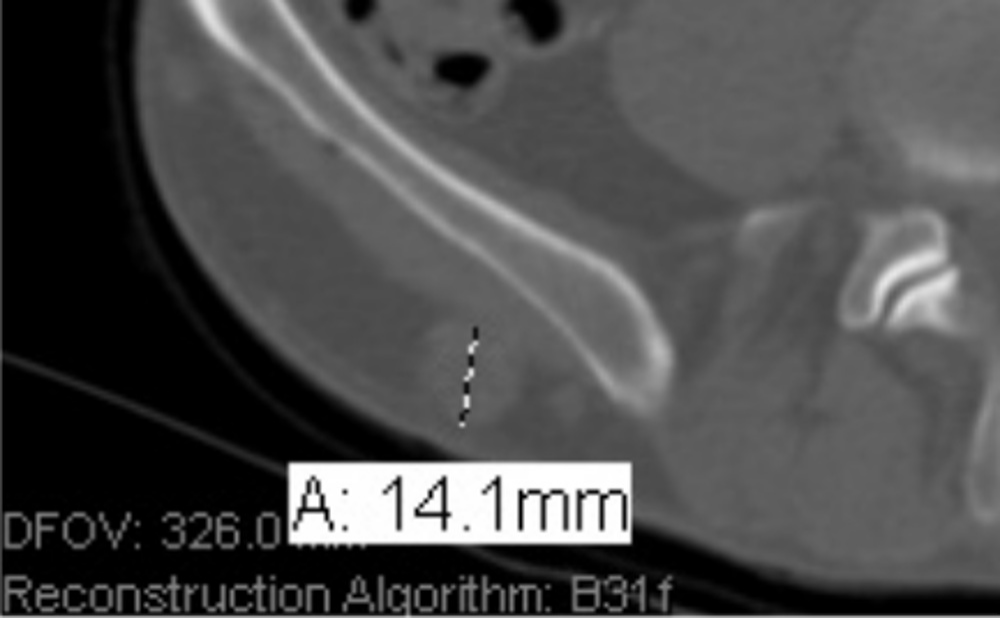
CT scan of the abdomen revealing deep tissue lesions concerning for neurofibroma.
